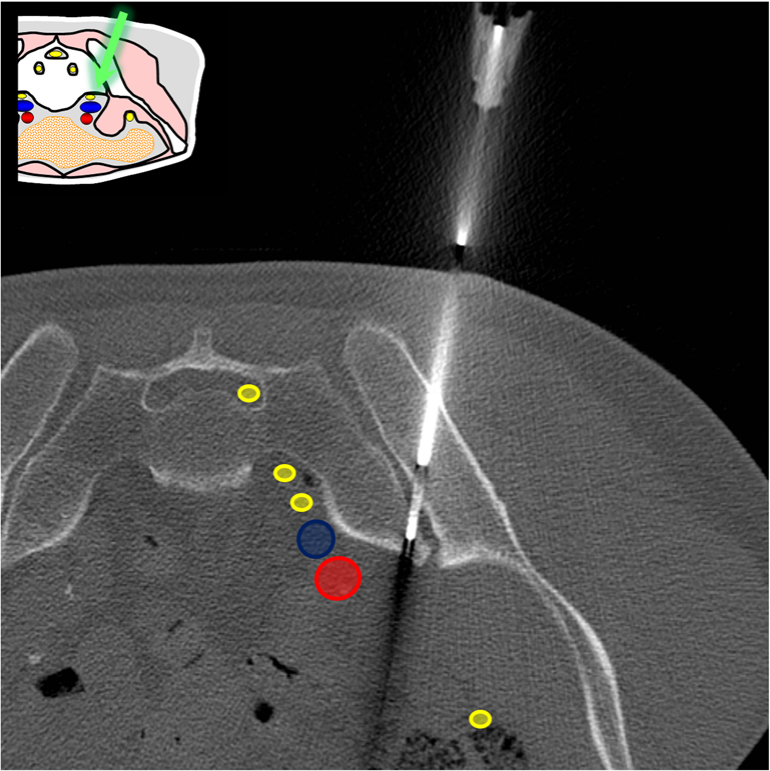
A 59-year-old man presenting with unilateral sacroiliitis, negative blood cultures and negative sacroiliac joint fluid aspiration. Axial CT shows the 11-G bone biopsy needle through the sacroiliac joint. This approach was planned to allow sampling of both the joint space and subchondral bone “sandwich technique” and increase biopsy yield
